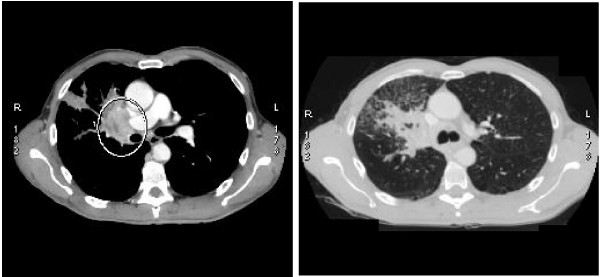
CT scan on June 2008.
